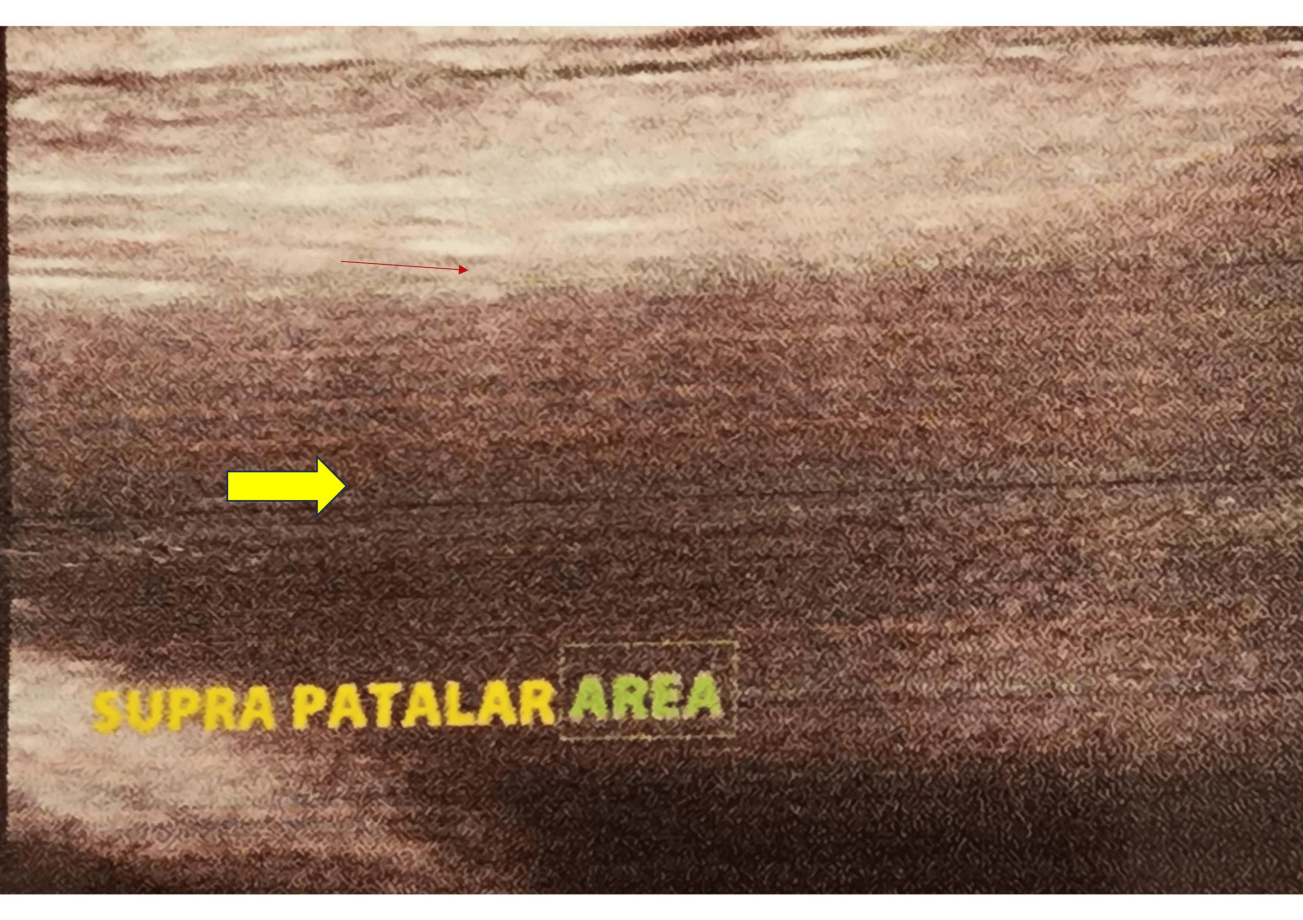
Ultrasonography (USG) of the knee joint showing fluid with synovial hypertrophy in the suprapatellar region The thick arrow indicates an anechoic area, and the thin arrow indicates synovial hypertrophy.
